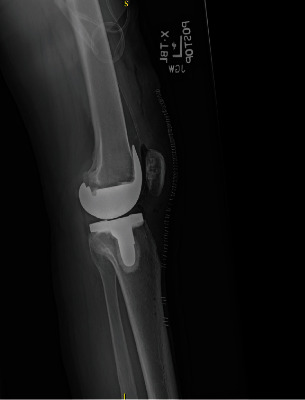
Postop lateral TKA X-ray.
